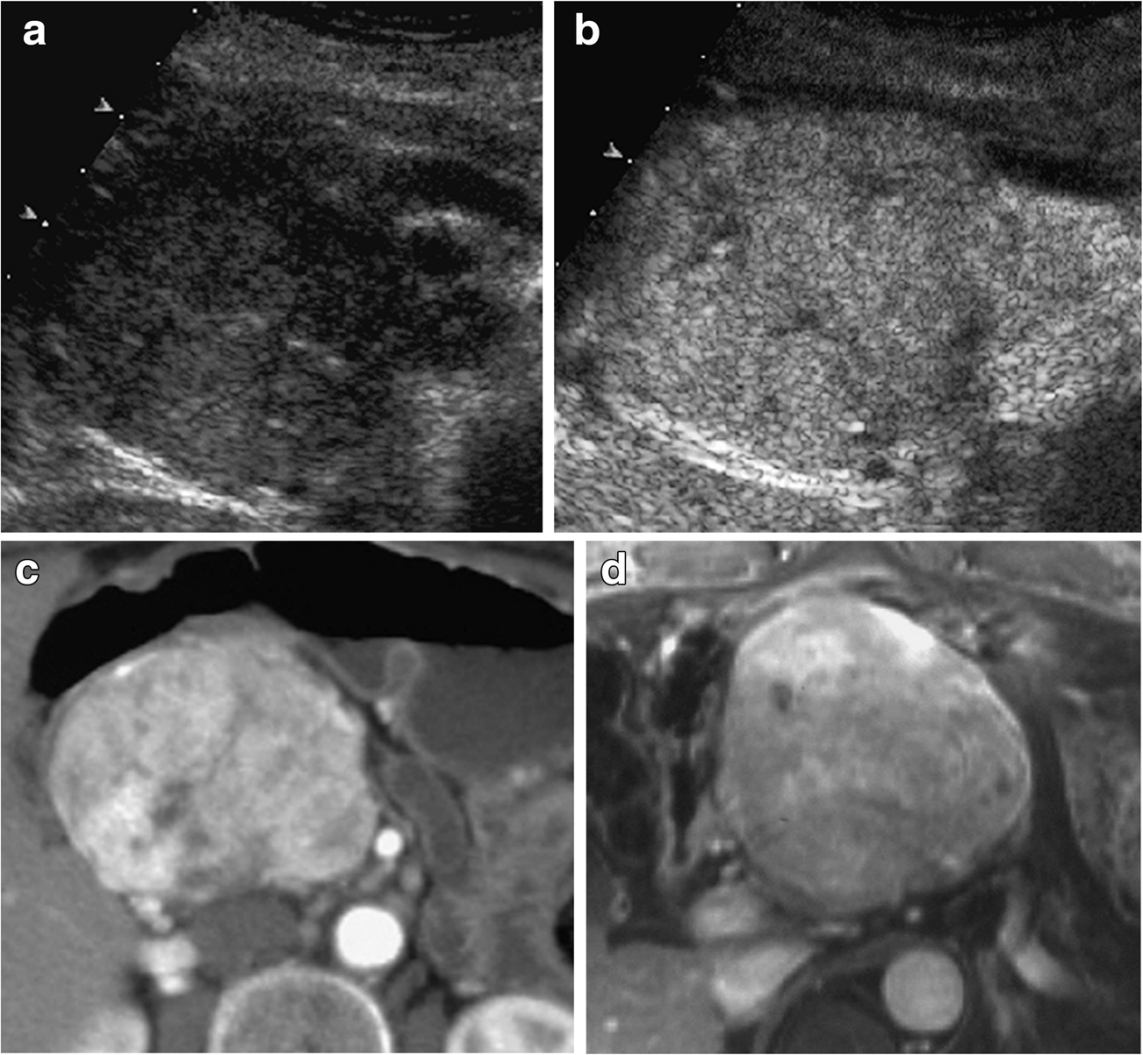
Non-functioning NEN. US and CEUS examinations: large hypoechoic mass (a) with small calcifications in the pancreatic head, causing upstream dilation of the Wirsung duct. This lesion is inhomogeneously hypervascularized at CEUS (b). CT examination: the pancreatic mass appears inhomogeneously hyperenhancing (c) in respect to the surrounding pancreatic parenchyma on dynamic phases. Dynamic MRI: inhomogeneous hypervascularity (d) of the pancreatic head mass
